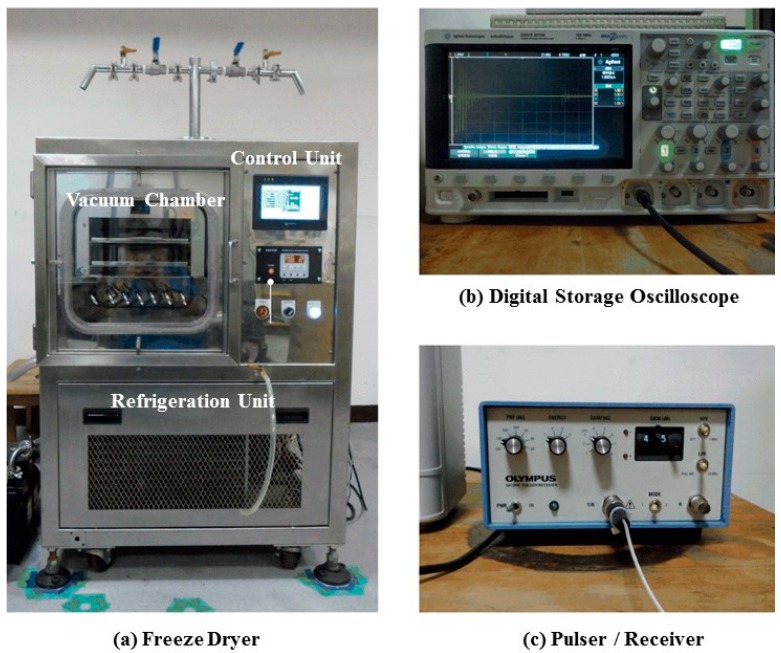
Photographs of (a) the freeze dryer machine with vacuum chamber, controller unit, refrigeration unit and vacuum pump; (b) the digital oscilloscope and (c) the ultrasonic pulser/receiver unit.
